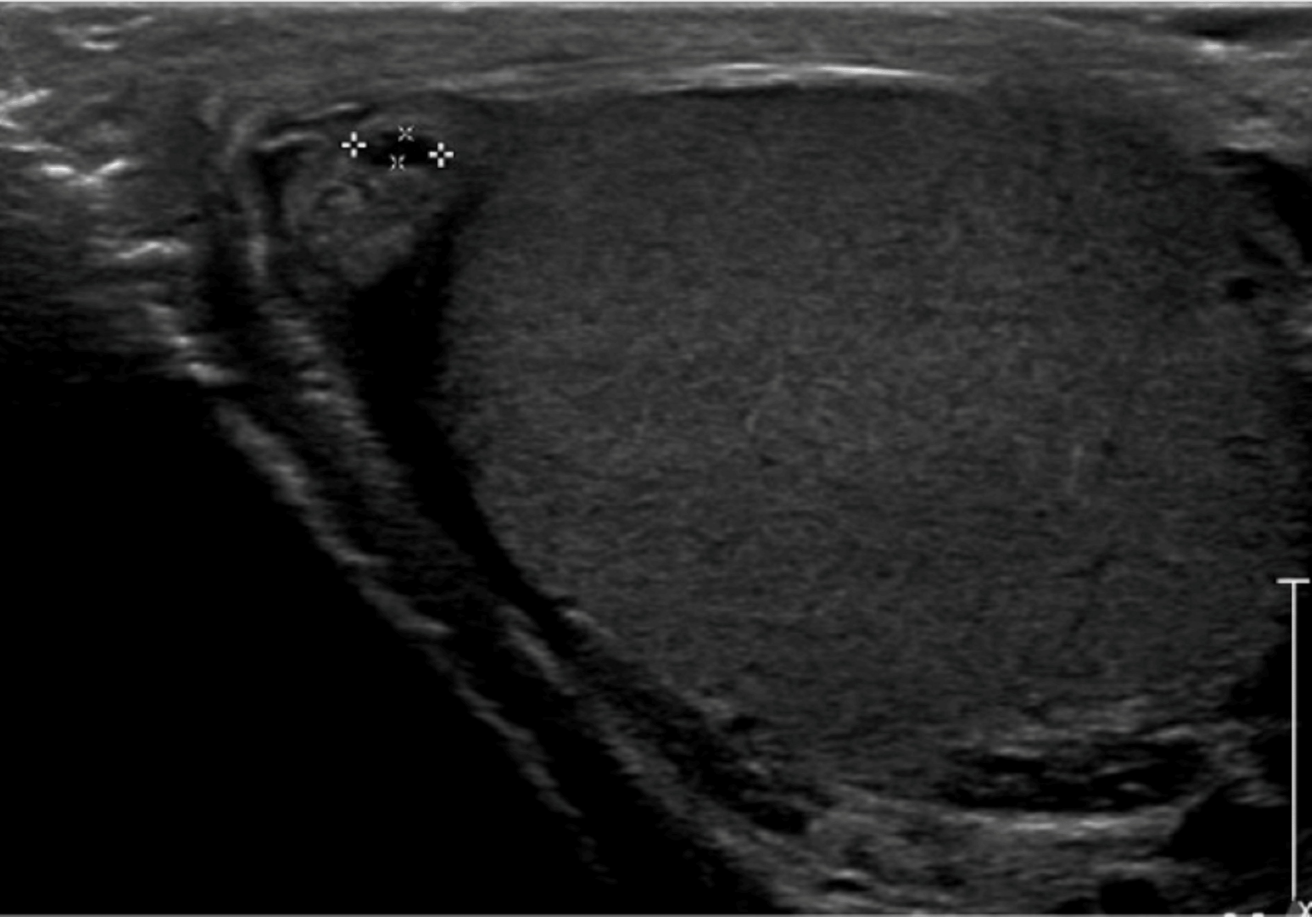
Ultrasound image showing a right epididymal head cyst.
